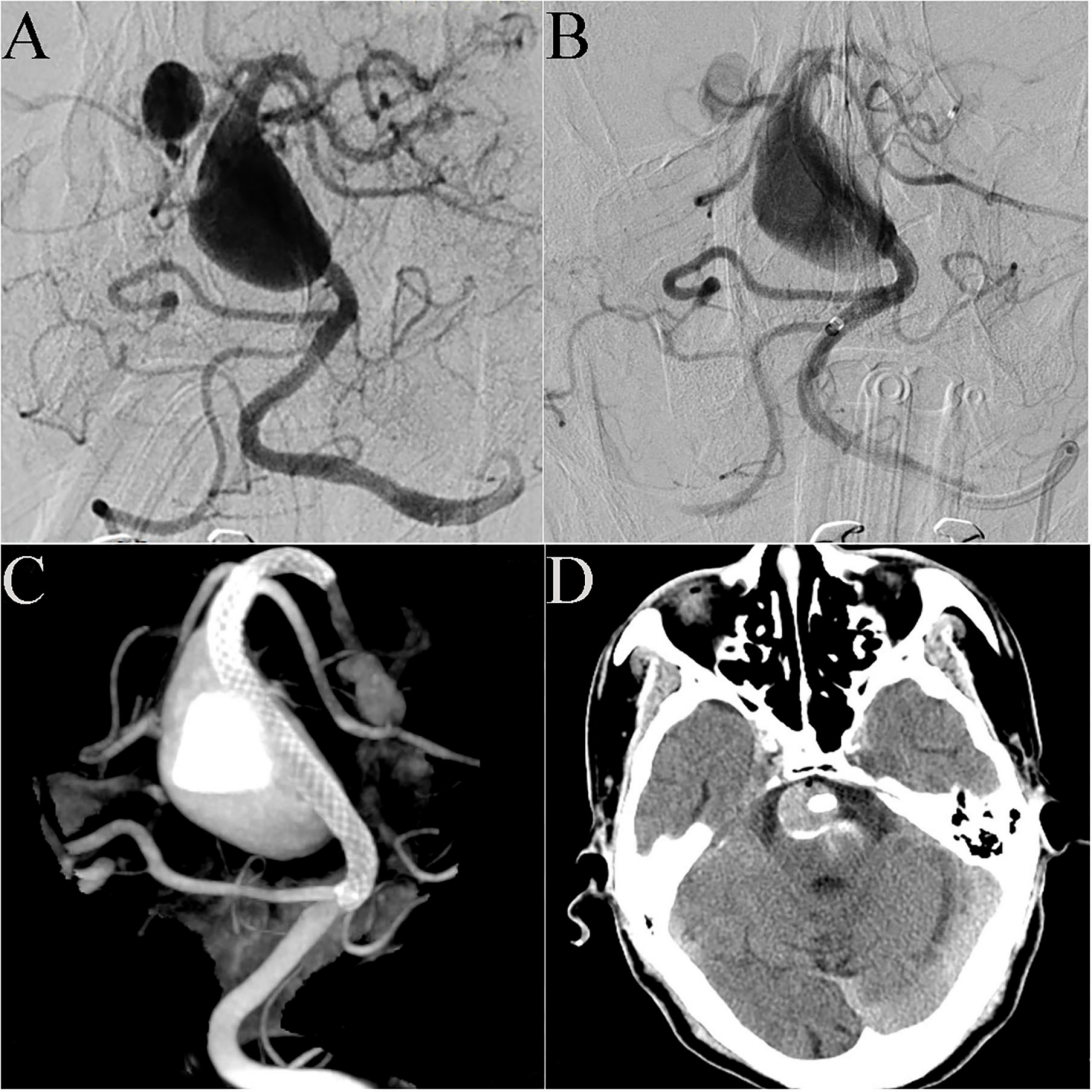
Case 20: DSA showed that the patient had a giant dissecting aneurysm of the basilar artery and a posterior cerebral arterial aneurysm (A). A 4.5 mm × 35 mm PED was successfully deployed in the basilar artery. Immediate post-embolization angiograms showed contrast stasis in the lumen of the aneurysm (B). Dyna CT showed good vascular reconstruction (C). Routine CT scanning showed no obvious bleeding or infarction on post-operative day 2 (D).
